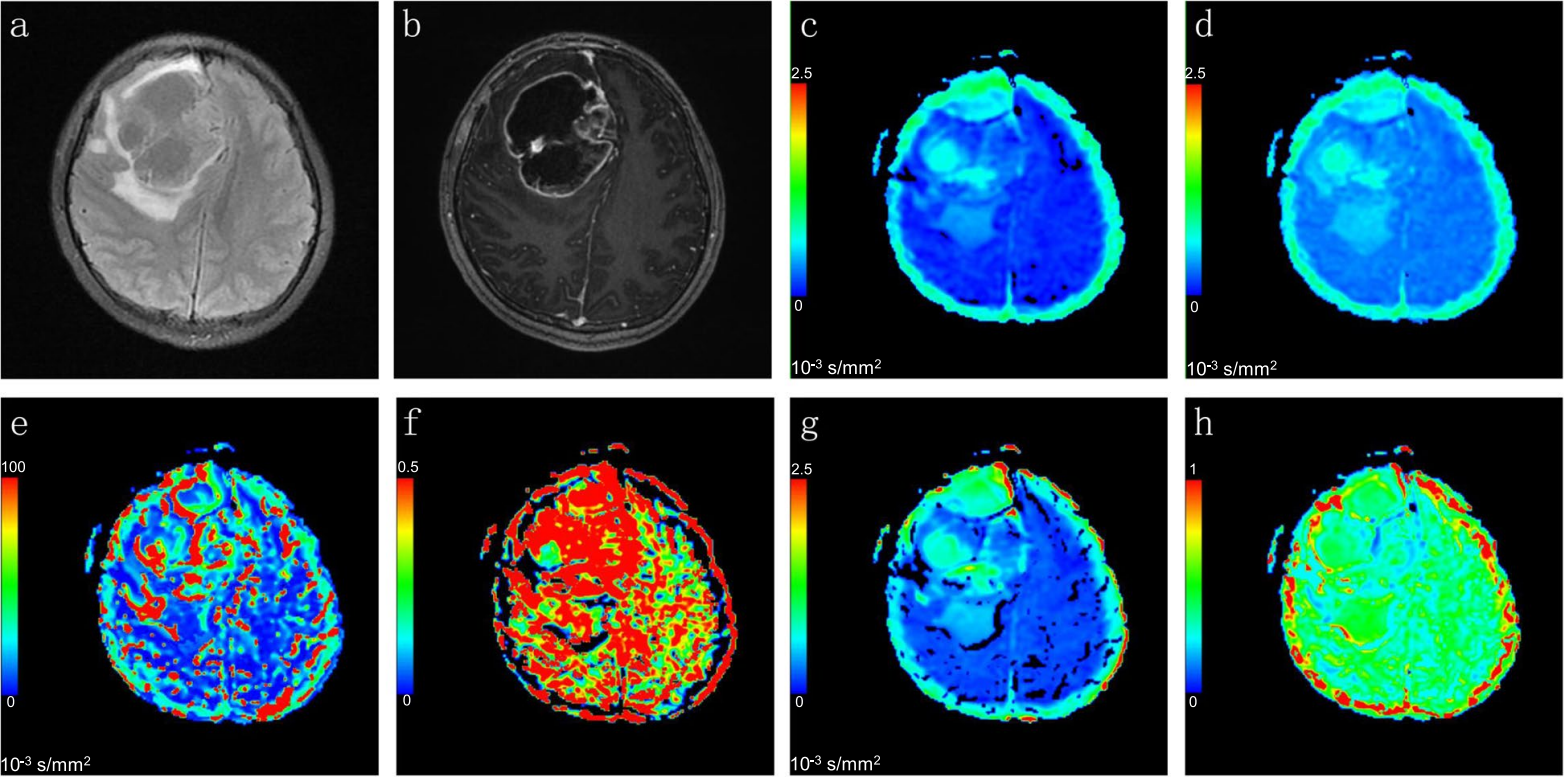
Representative images of a patient with tumour progression. Axial FLAIR (a) and contrast-enhanced T1WI (b) demonstrated a newly enhanced lesion in the right frontal lobe. The lesion grew after six cycles of temozolomide chemoradiation, implying that the lesion had progressed. The ADC (c), D (d), D* (e), f (f), DDC (g) and α (h) maps were calculated automatically from the MADC software
